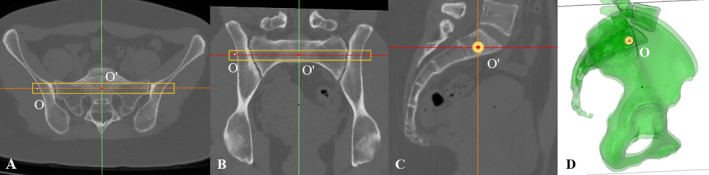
A 22‐year‐old female patient suffered pelvic anterior ring injury and intact posterior ring. The CT images were recut to obtain the strict axial plane (A), the strict coronal plane (B), and the strict mid‐sagittal plane (C). The cylinder (yellow rectangle) represented the most sufficient osseous corridor for a transiliac‐transsacral screw. Point O (white) was the intersection of the cylinder's axis and the outer iliac cortex, indicating the optimal entry point. Point O′ (red) was the intersection of the cylinder's axis and the strict mid‐sagittal plane. Point O and O′ were overlapped on the lateral view of the 3D pelvis (D).
